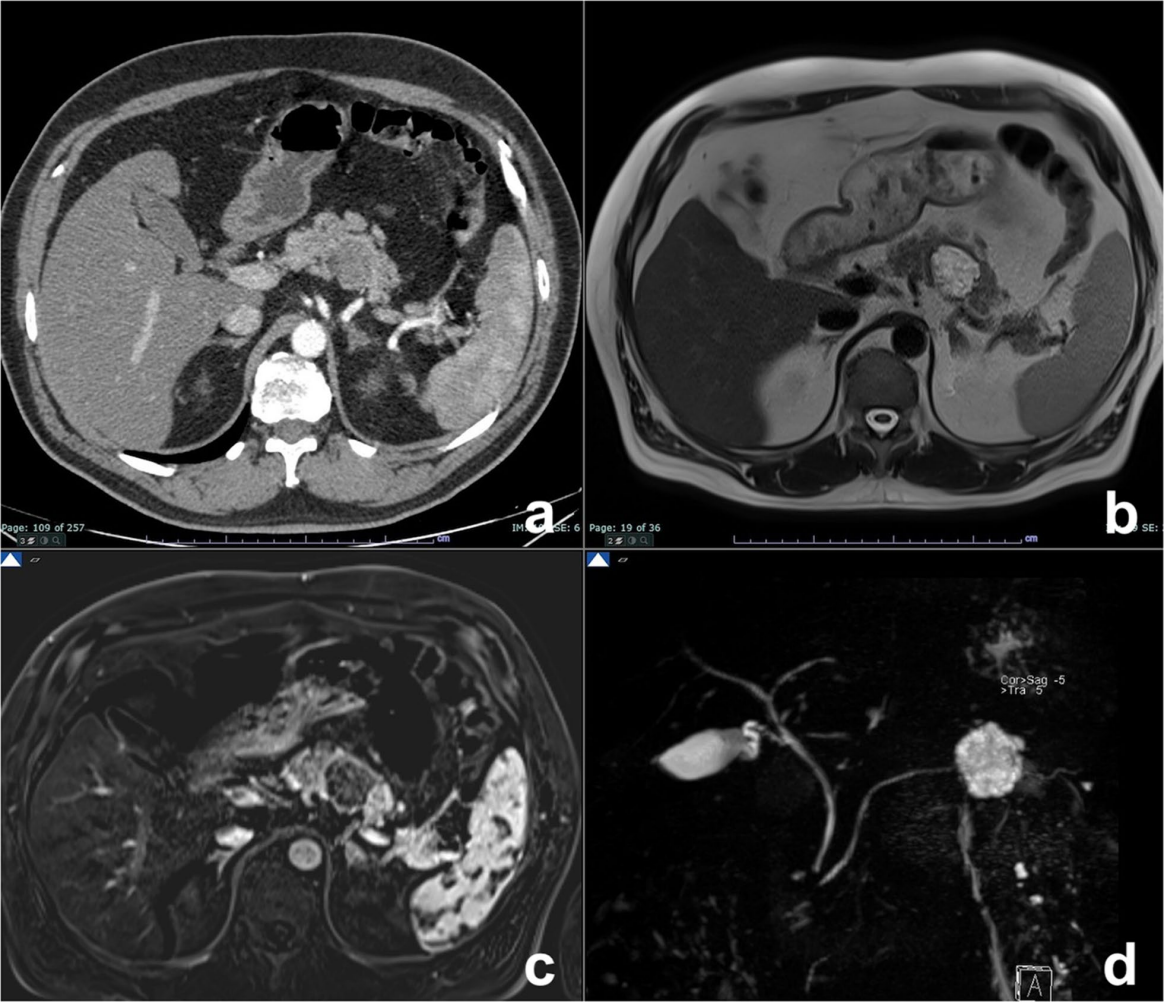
Serous cystadenoma, “honeycomb” pattern. On contrast enhanced CT (a), a large hypodense lesion with tiny central enhancement can be appreciated in the body–tail of the pancreas. On T2w MRI, the lesion is hyperintense (b). On T1w dynamic imaging, the central enhancement is appreciable, especially with subtraction imaging (c), related to the enhancement of septa and stroma supporting the microcysts. MRCP (d)
